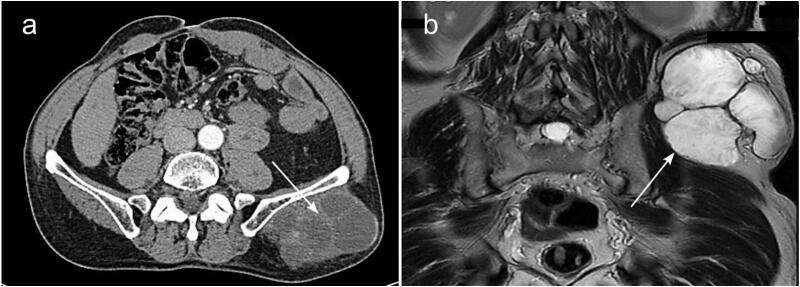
Pre-operative imaging findings. a: Abdominal CT scan with contrast, transverse section passing through the mass (White arrow). b: Coronal view of T2 weighted MRI of the soft tissues showing a cluster of cystic lesions embedded in the subcutaneous fat of the lumbar region (White arrow).
